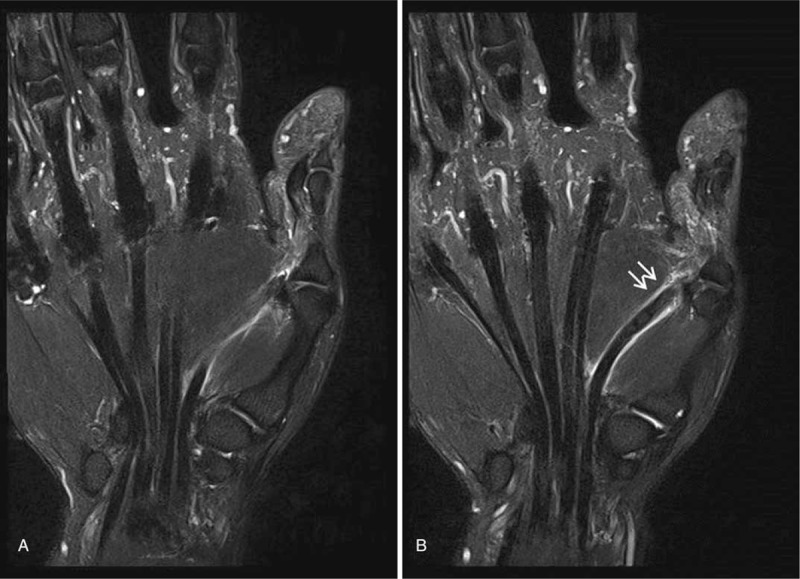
(A, B) Coronal T2-weighted MRI of the left thumb, showing complete FPL rupture at the level of the distal one-third of the proximal phalanx; the proximal end of the ruptured tendon was retracted to the level of the MP joint (arrows).
